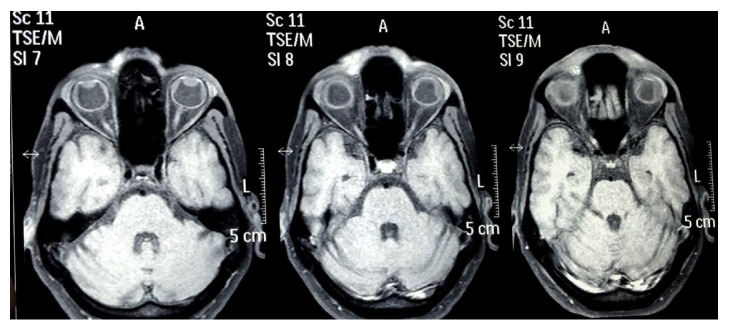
Axial T1 weighted MRI of head and orbit showing bilateral choroidal detachment with bilateral suprachoroidal haemorrhage.
